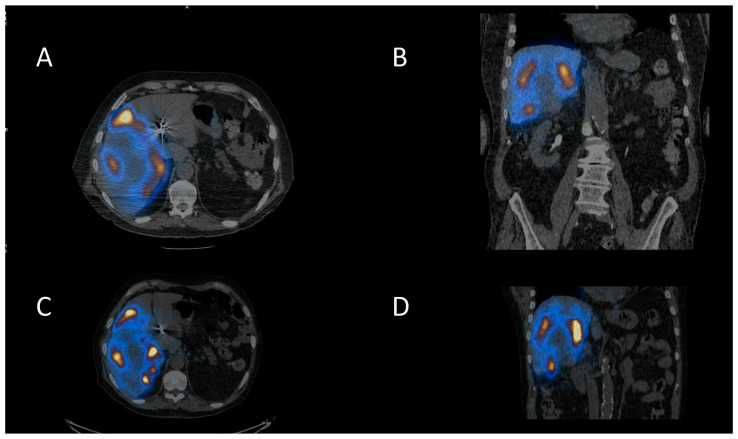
Selective internal radiotherapy with radiolabelled microspheres for liver metastases. (A) Axial and (B) coronal fused SPECT CT images of ^99m^Tc-MAA (macroaggregated albumin) pre-treatment imaging for dose calculation and treatment verification. Corresponding (C) axial and (D) coronal post-therapy PET CT images following therapy with ^90^Y-microspheres.
